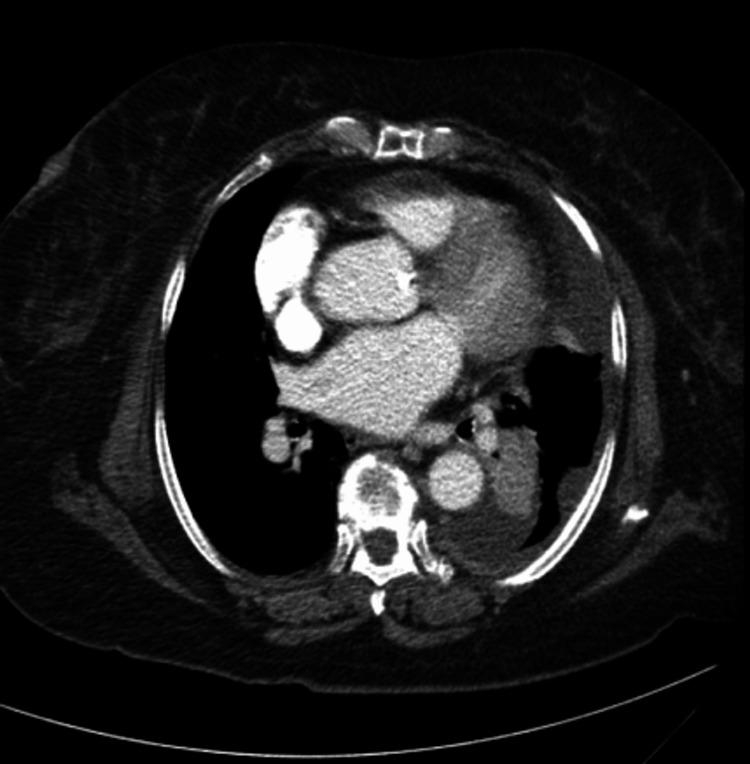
CT scan showing left-sided loculated pleural effusion and pleural thickening.
